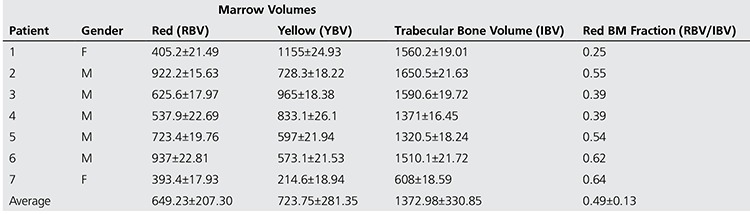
Estimated Whole Body BM volumes using 18F-FDG PET/CT imaging
